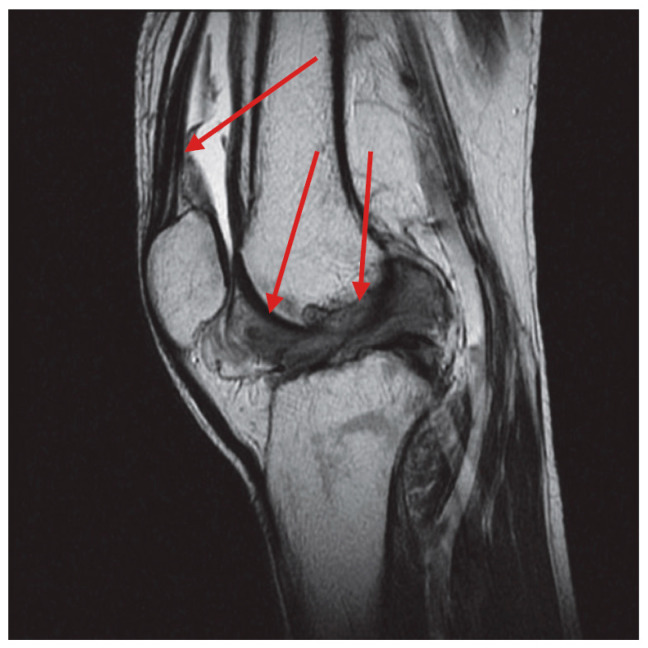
T2-weighted magnetic resonance sagittal scan of a D-TGCT. The location of disease is indicated by red arrows.
